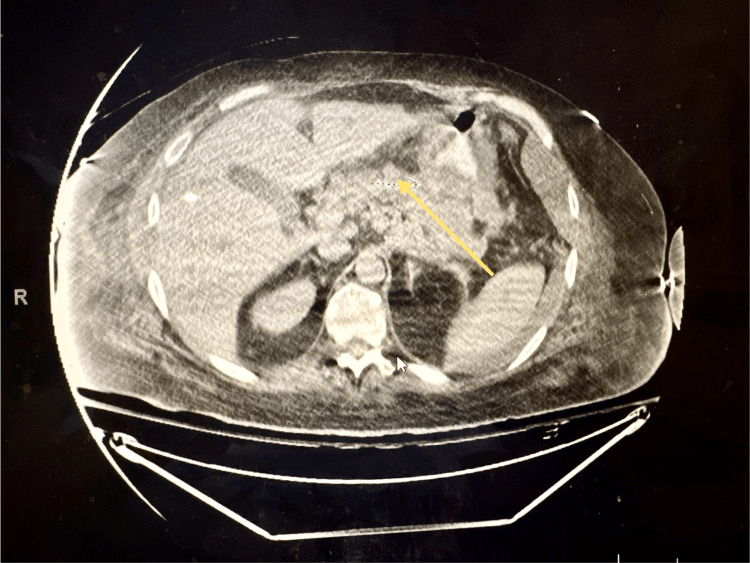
CT scan of Abdomen This imaging shows areas very small low attenuation within the pancreatic parenchyma, indicating necrosis, accompanied by high attenuation areas representing blood collections within the pancreas and surrounding tissue, signifying hemorrhage
